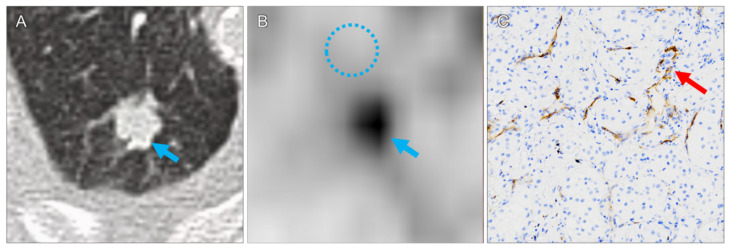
Pulmonary metastasis in the right upper lobe ((A), arrow; lesion 6). Due to the low background activity in the lung ((B), circle, SUVmax 0.9) the moderate tracer uptake in the tumor is clearly visible ((B), arrow, SUVmax 4.2). Moderate microvascular PSMA expression ((C), arrow).
